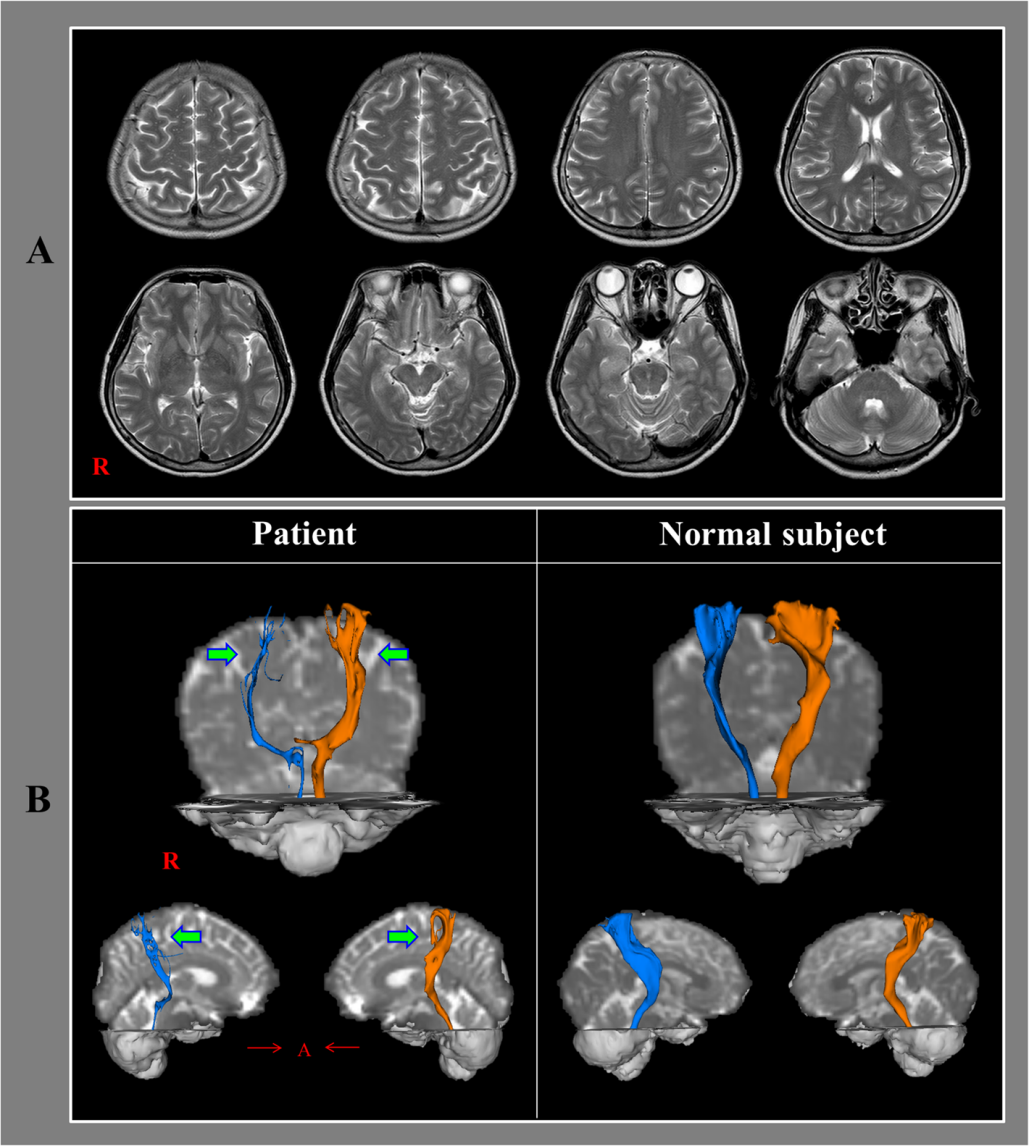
a T2-weighted brain magnetic resonance images taken at 4 years after onset shows no definite lesion. b Results of four-year post-trauma diffusion tensor tractography. In both hemispheres, the spinothalamic tract (STT) shows partial tearing in the upper portion (arrows) compared with those in a normal control (51-year-old female)
